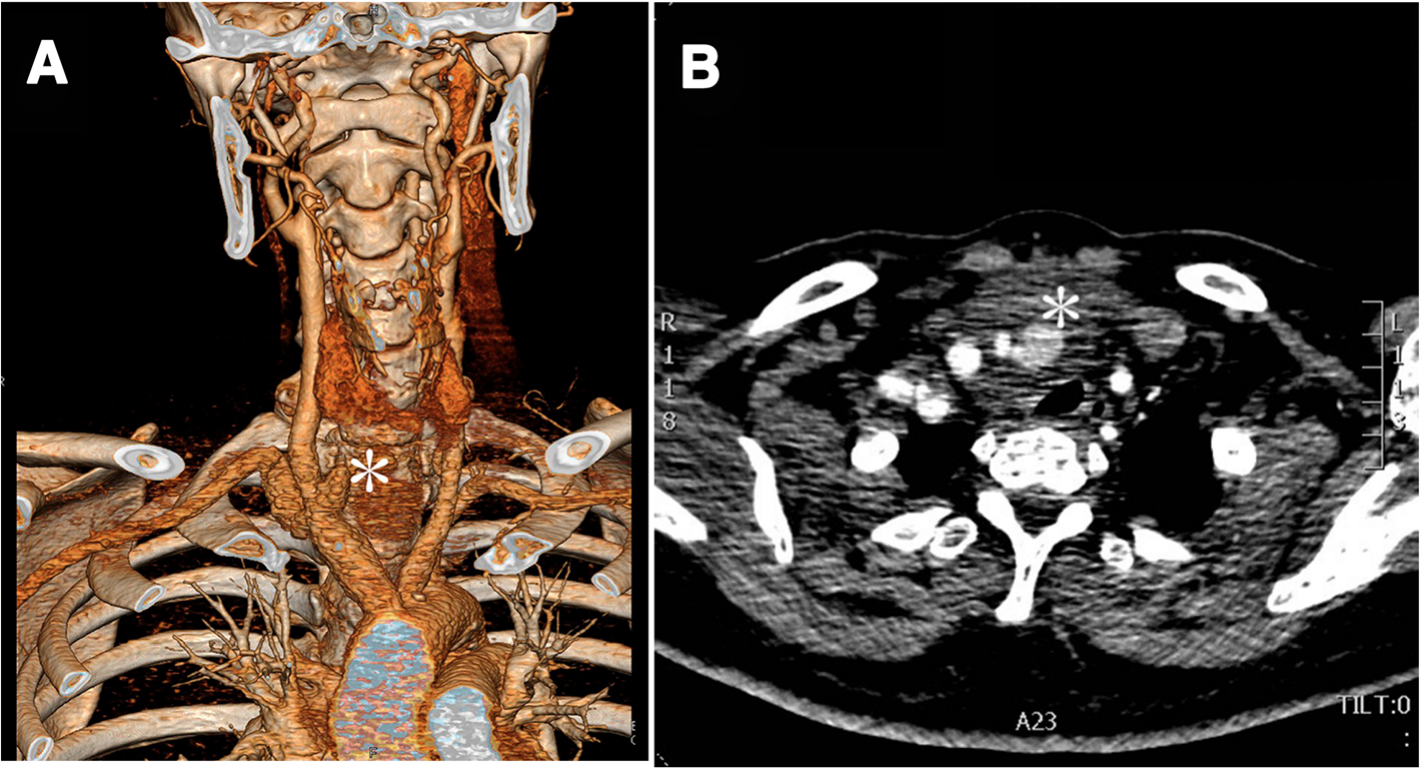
a Computed tomography angiography shows that the rupture area is located on the bifurcation of the innominate artery. *Star mark* (*) shows the position of the pseudoaneurysm. b Transverse computed tomography slide shows the pseudoaneurysm (*white star mark ); trachea is compressed slightly by the pseudoaneurysm
